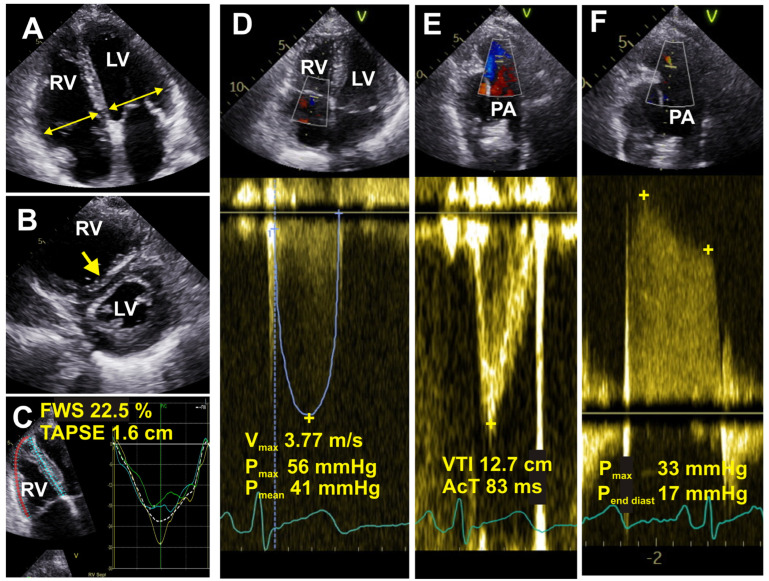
Echocardiographic images are shown in a patient with idiopathic pulmonary fibrosis. (A) Four chamber view: the maximum diameters of the right (RV) and left (LV) ventricles are measured (yellow arrows). Dilated RV with a ratio to left ventricle at about 1.0 is shown. (B) Parasternal short-axis view: large RV, flattened intraventricular septum (yellow arrow). (C) Right ventricular speckle strain, four chambers adjusted for RV: tricuspid annular plane systolic excursion (TAPSE) is reduced at 1.6 cm, and free wall right ventricular strain (FWS) is also reduced at 22.5%. (D) Four chamber view: septal deviation towards LV showing evidence of elevated RV pressure. Continuous wave Doppler through the tricuspid valve and Vmax is elevated at 3.77 m/s. (E) Pulse wave Doppler through right ventricular outflow tract (RVOT) indicating shortened pulmonary artery (PA) acceleration time (AcT) at 83 ms. (F) Continuous wave Doppler: PA regurgitation, estimated end diastolic PA pressure is elevated at 17 mmHg.
